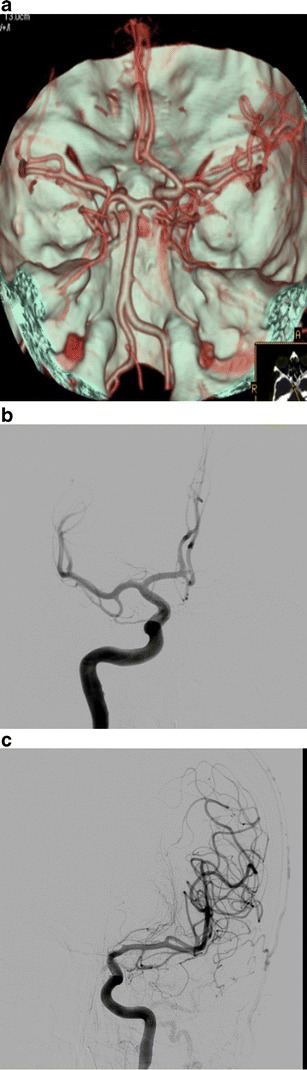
a CTA (VRT 3D reconstructions) reveals aplasia of the left A1 segment of the anterior cerebral artery in a patient with an Acom aneurysm. b Digital subtraction angiography (*AP view*) of the right ICA verifies the presence of a small Acom aneurysm. Both A2 segments are opacified from this single injection. c Digital subtraction angiography (*AP view*) of the left ICA. The left A1 segment is absent
